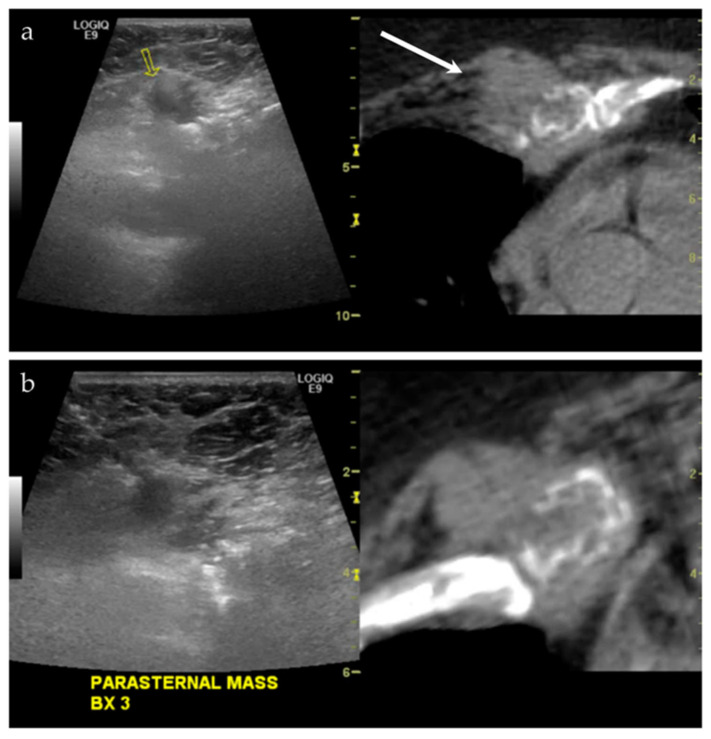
A middle-aged male with a history of lung and laryngeal carcinoma. The patient presented with a palpable lump at the left chest. (a) Ultrasound and the CT fused image shows a mass-like lesion at the left parasternal region, causing destruction of the chondral cartilage of the adjacent rib. This is outlined by a yellow arrow on the left image from the imaging software, and a white arrow on the right image. This lesion is considered “difficult to access” because of the pericardial fat and pericardium that are located just posterior to the lesion. (b) Targeted biopsy, with the use of color Doppler imaging (not shown), was successful. Final pathology showed metastatic disease from lung adenocarcinoma.
